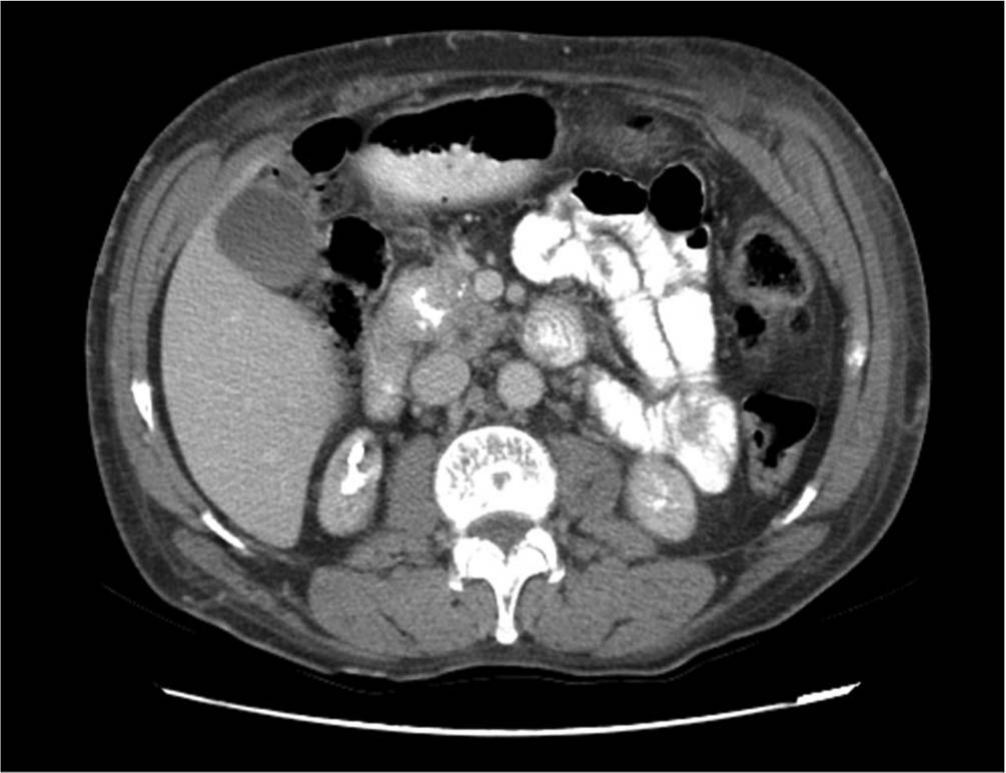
Triphasic computed tomography of the pancreas, portal phase, showing a tumor in the head of the pancreas.
